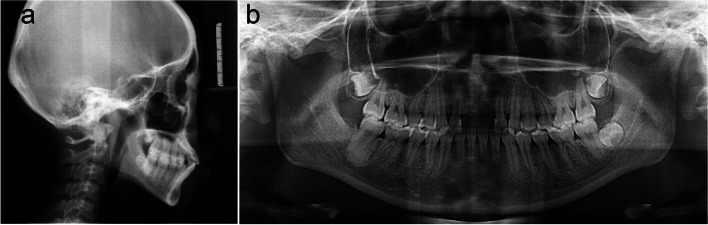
a Pretreatment radiographs: lateral cephalogram. b panoramic radiograph
